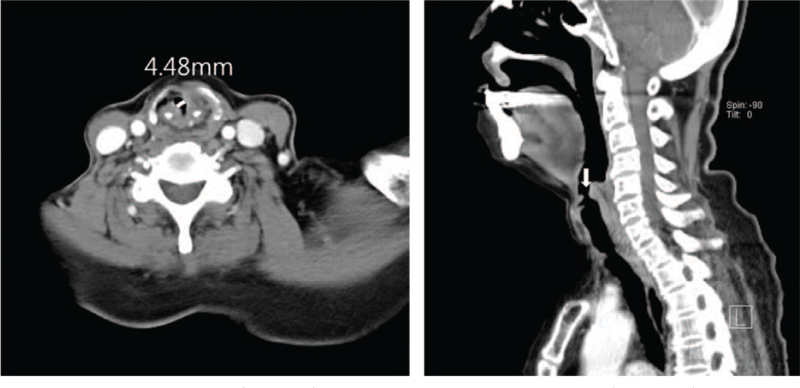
Preoperative axial computed tomography view (left, arrow), and sagittal computed tomography view (right, arrow) showing a very small opening into the larynx (supraglottic obstruction).
